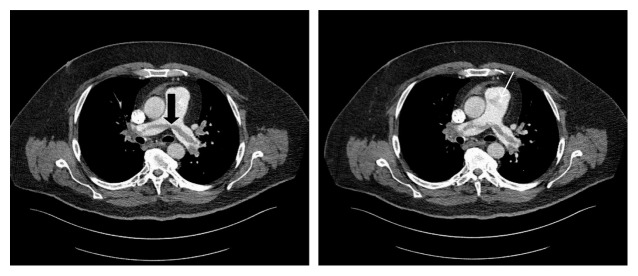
Computed Tomographic Pulmonary Angiogram (CTPA) showing massive saddle pulmonary embolus (bold arrow) with hypodense area at the root of pulmonary artery consistent with other emboli (white arrow).
